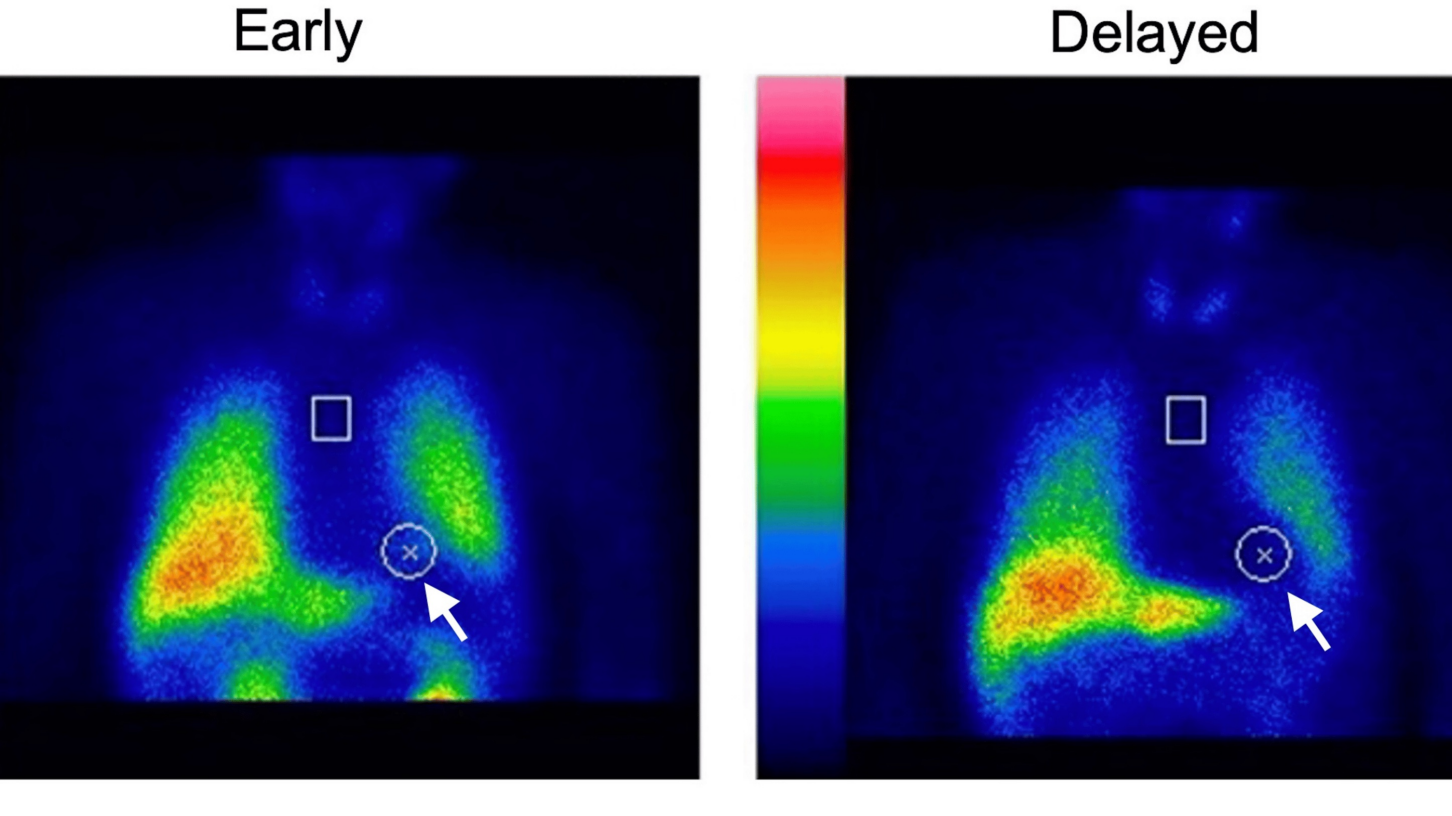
123I-MIBG myocardial scintigraphy The image shows reduced accumulation in the heart (white arrows), with heart-to-mediastinum (H/M) ratios of 1.84 (early) and 1.52 (delayed), and a washout rate of 43.4%. 123-I MIBG: 123 Iodine-metaiodobenzylguanidine.
